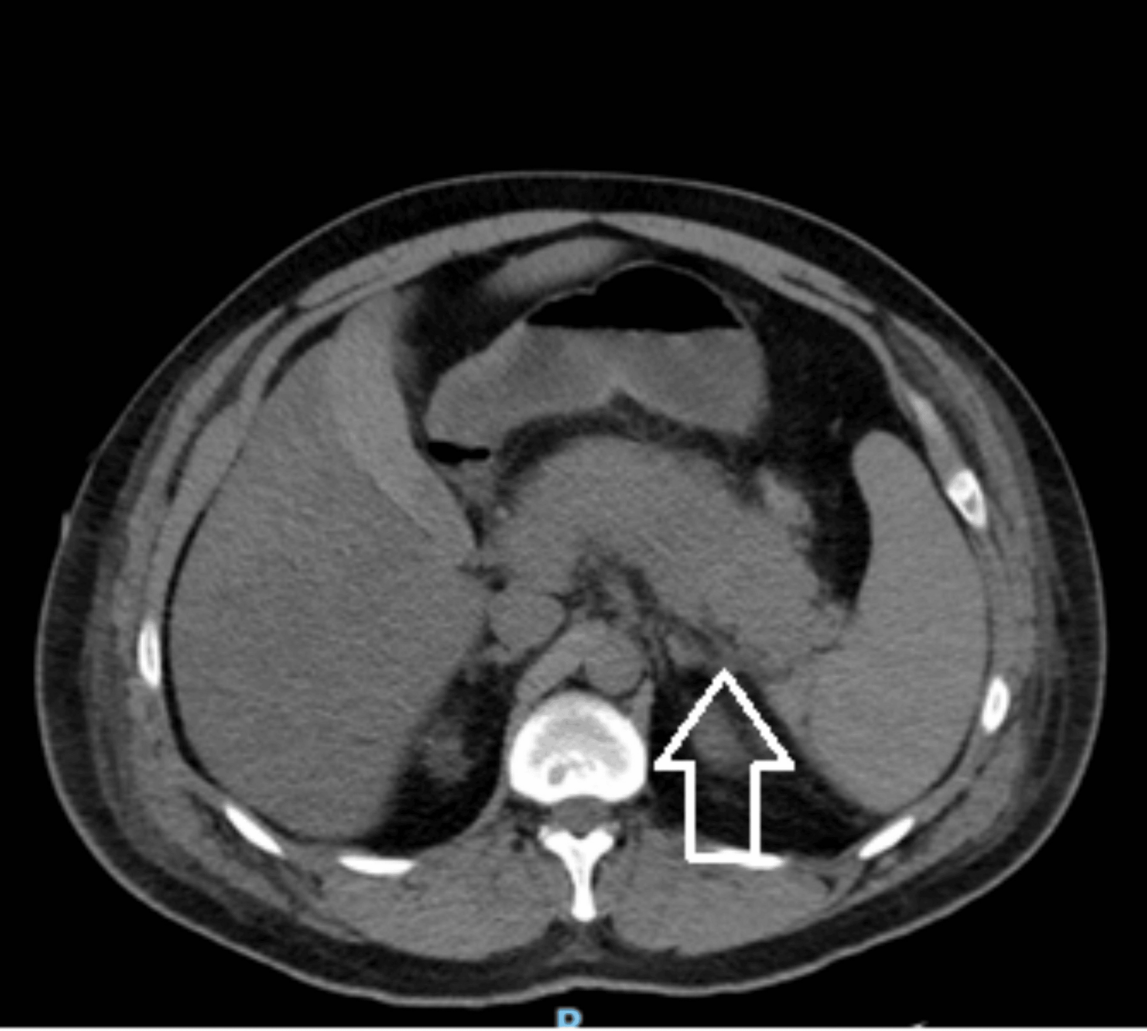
Follow-up contrast-enhanced CT of the abdomen and pelvis on hospital day four Representative axial CT image from serial imaging demonstrating evolving pancreatitis-related inflammatory changes during hospitalization. The arrow indicates an area of peripancreatic inflammatory change.
